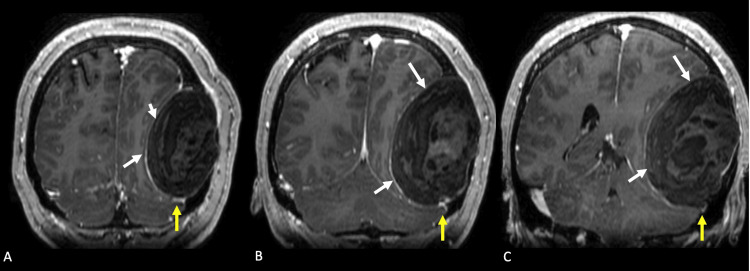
Coronal post-contrast T1-weighted images (A-C) demonstrating transverse sinus (yellow arrows) and suppression of the tentorium.
